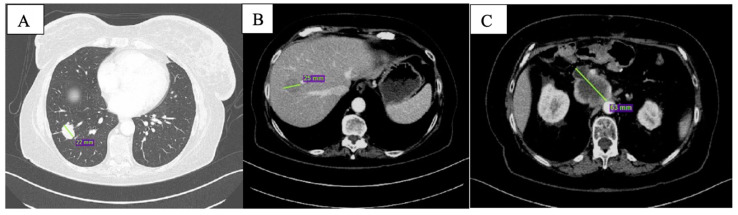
Baseline CT scan showing nodular lesion in inferior lobe of the right lung (A), hepatic lesion in the 8th segment (B) and peripancreatic tissue (C).
